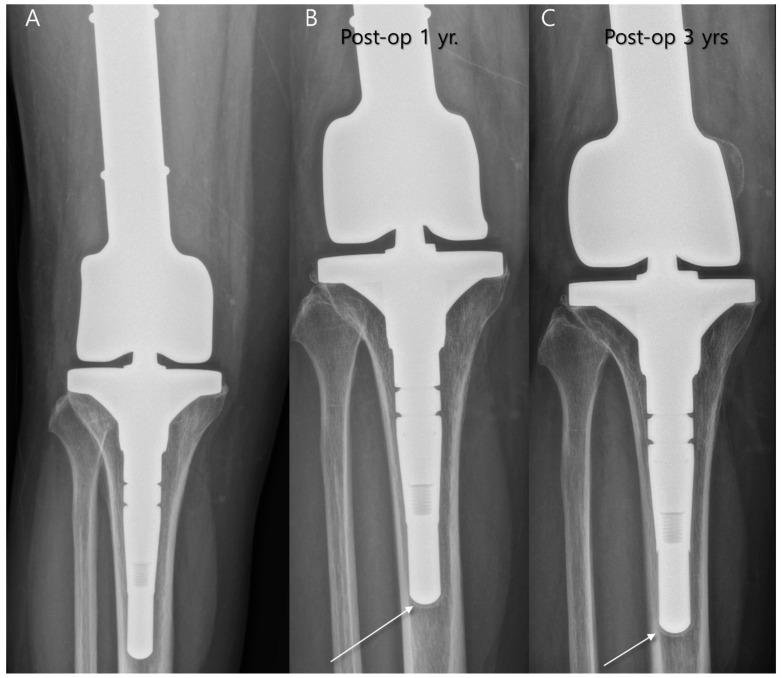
Endoprosthetic replacement at the distal femur due to malignancy in giant cell tumor. (A) Immediate postoperative plain radiograph is shown. (B) One-year follow-up plain radiograph shows a thin radiolucent zone at the metal–bone interface, measuring less than 2 mm (arrow). (C) Three-year follow-up plain radiograph shows no progression (arrow), indicating fibrous tissue ingrowth rather than implant loosening.
